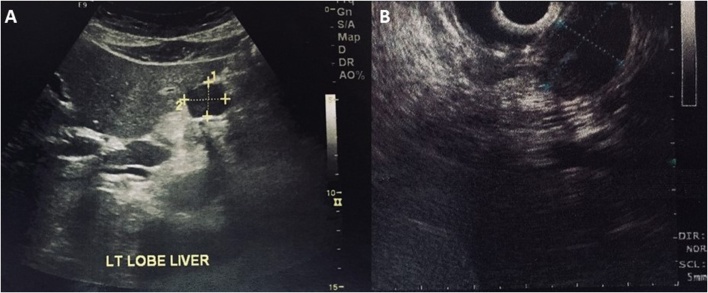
A: Ultrasonography scan of the upper abdomen shows a round and well-defined mass in the stomach. Fig. 1B: Endoscopic ultrasound reveals a 2.5 × 1.5 cm hypoechoic mass that appears to arise from the muscolaris propria of the lesser curvature.
